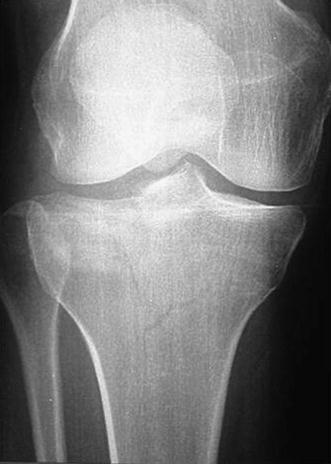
Schatzker type V fracture, pre-op X-rays (AP)
